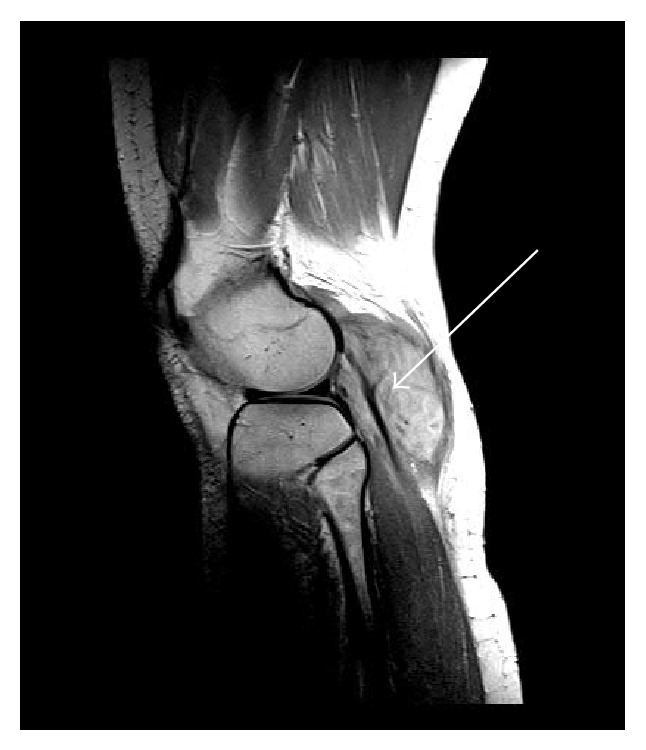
MRI scan, sagittal view.
